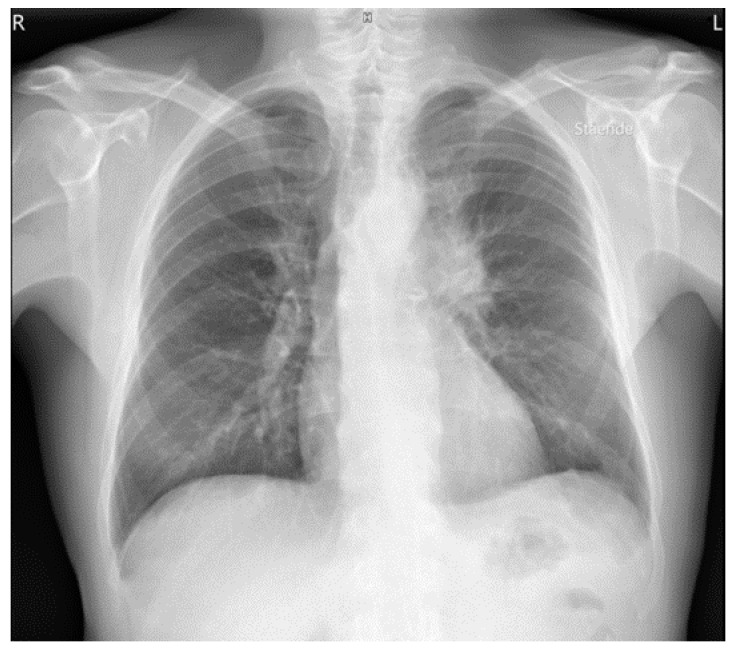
Chest X-ray with a large left hilar mass.
